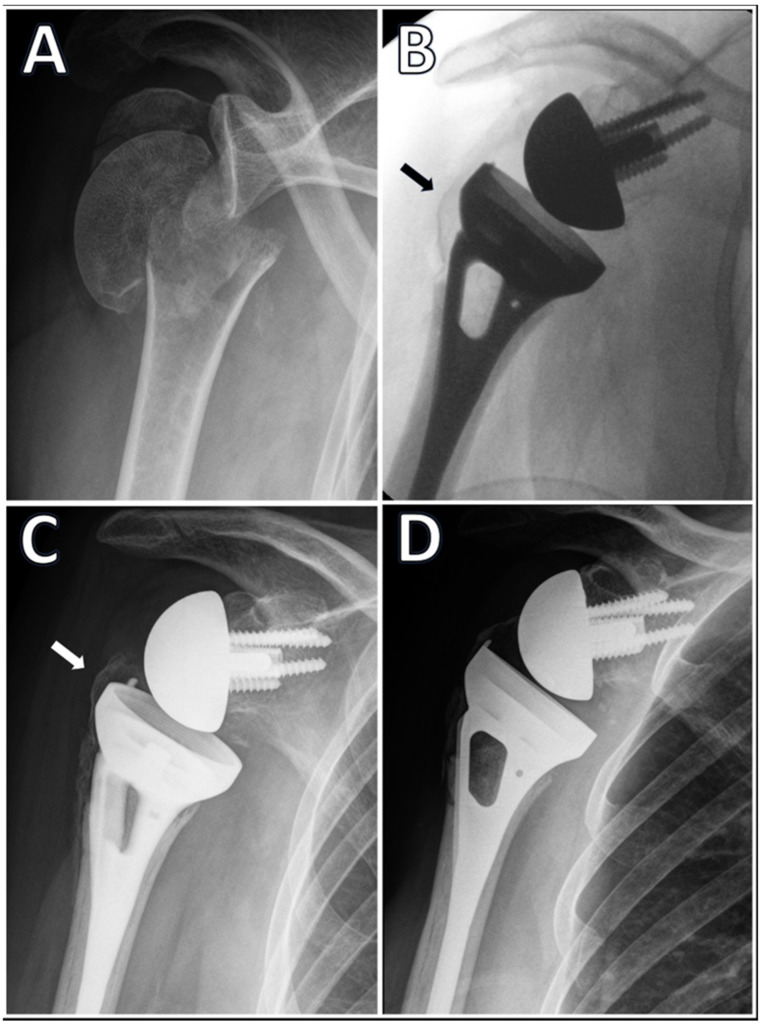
A 73-year-old female presented with a 3-part displaced fracture of the proximal humerus (A); she underwent RSA using Tornier Aequalis reversed fracture prosthesis. Intraoperative fluoroscopy shows a good reduction in the greater tuberosity (black arrow) (B); an AP radiograph taken 6 weeks after surgery shows the proximal migration of the greater tuberosity (white arrow), which now overhangs the tray of the prosthesis, and is not in continuity with the shaft (C); at 3.5 months, the greater tuberosity is almost completely absorbed and is not considered united (D).
